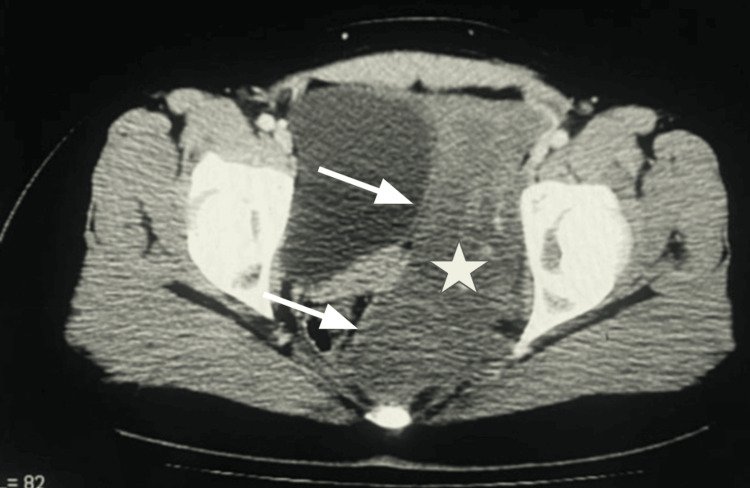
Axial contrast-enhanced CT of the pelvis demonstrating the lesion extending into the left ischiorectal fossa and perineum, without evidence of invasion into the adjacent rectum or bladder. Star highlights the center of the mass. Arrows point to the boundaries of the bladder and rectum.
